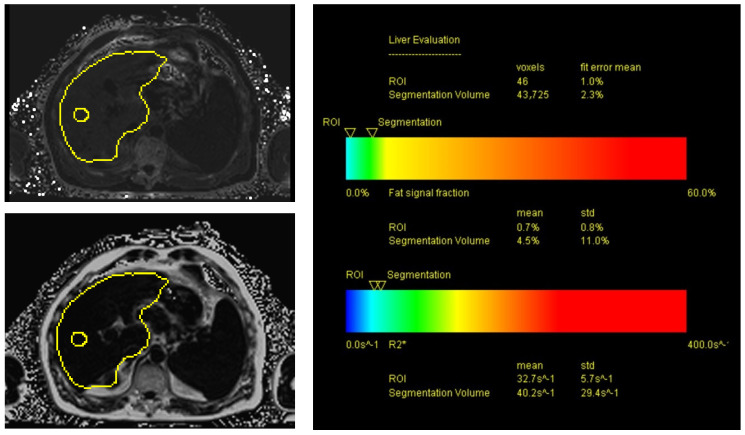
MRI PDFF sequences in axial view of a patient with known liver cirrhosis. The fat signal fraction inside the positioned ROI (see yellow ROI in left images) showed mean values <5%, consistent with no evidence of hepatic steatosis.
